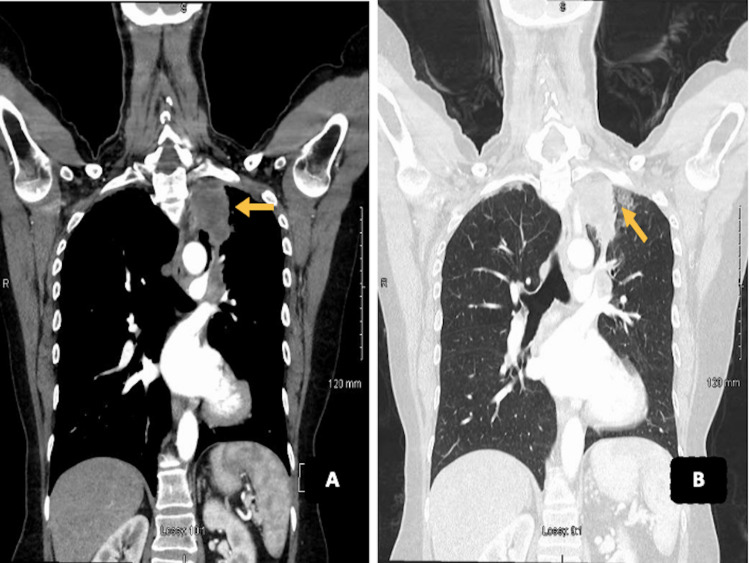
CT of the neck and thorax with contrast (A) Intravenous (IV) contrast-enhanced CT of the neck and thorax and (B) IV with multiplanar reconstruction showing a 5.4 x 4.1 x 6 cm left apical mass, which is associated with volume loss of the left upper lobe, suspicious for a Pancoast tumour.
